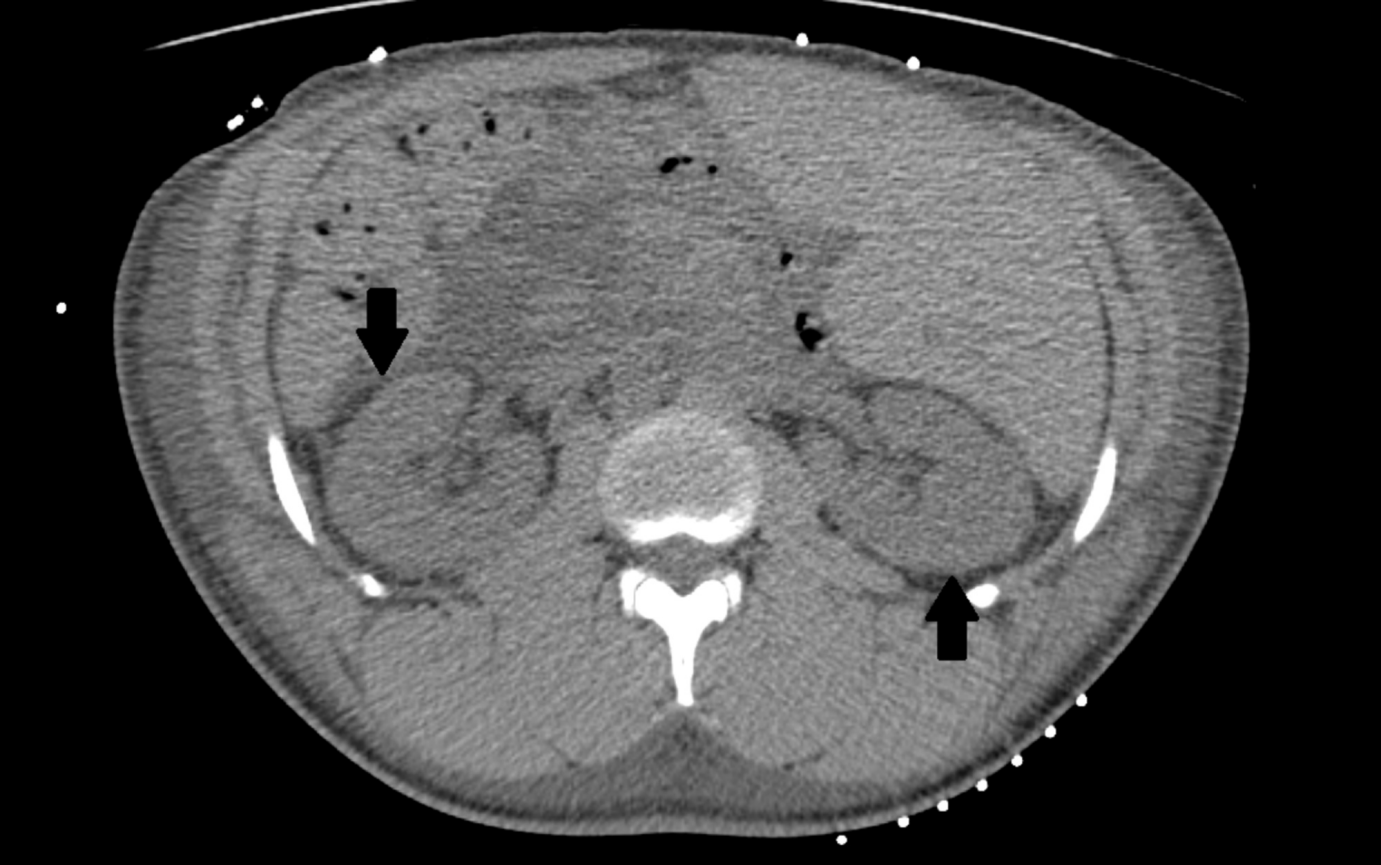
CT scan abdomen and pelvis Black arrows point towards increased echogenicity in bilateral renal cortex.
